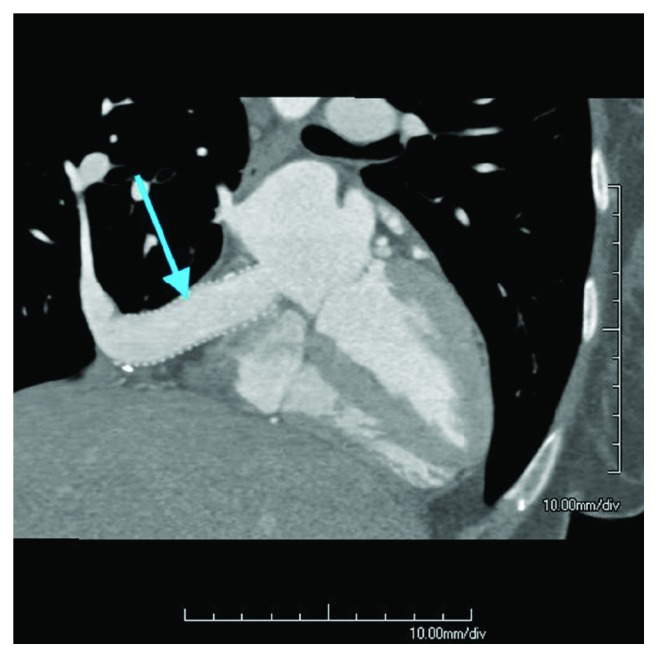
Oblique multiplanar reformat of postoperative CTA. Arrow pointing to the widely patent artificial baffle connecting the scimitar vein with the left atrium via the posterior aspect of the right atrium. There is no filling defect inside the graft to suggest thrombus.
